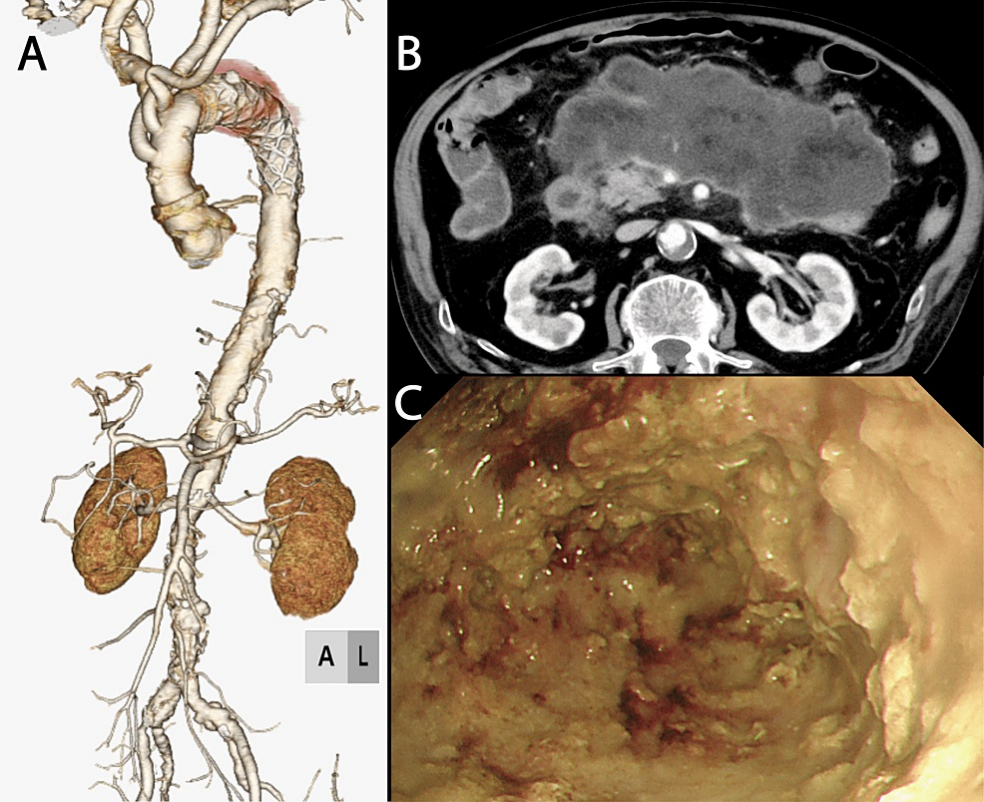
Computed tomography imaging after operation. (A) Volume rendering image. The aortic arch aneurysm was resected and the frozen elephant trunk was placed at the aortic arch and descending aorta. (B) An axial image at the pancreas level indicated a huge, infected pancreatic necrosis. (C) An image of the necrotic pancreas being debrided via gastrointestinal endoscopy. This is an image of the pancreas. Extensive necrotic tissue has been removed, exposing a pancreas that appears close to normal.
